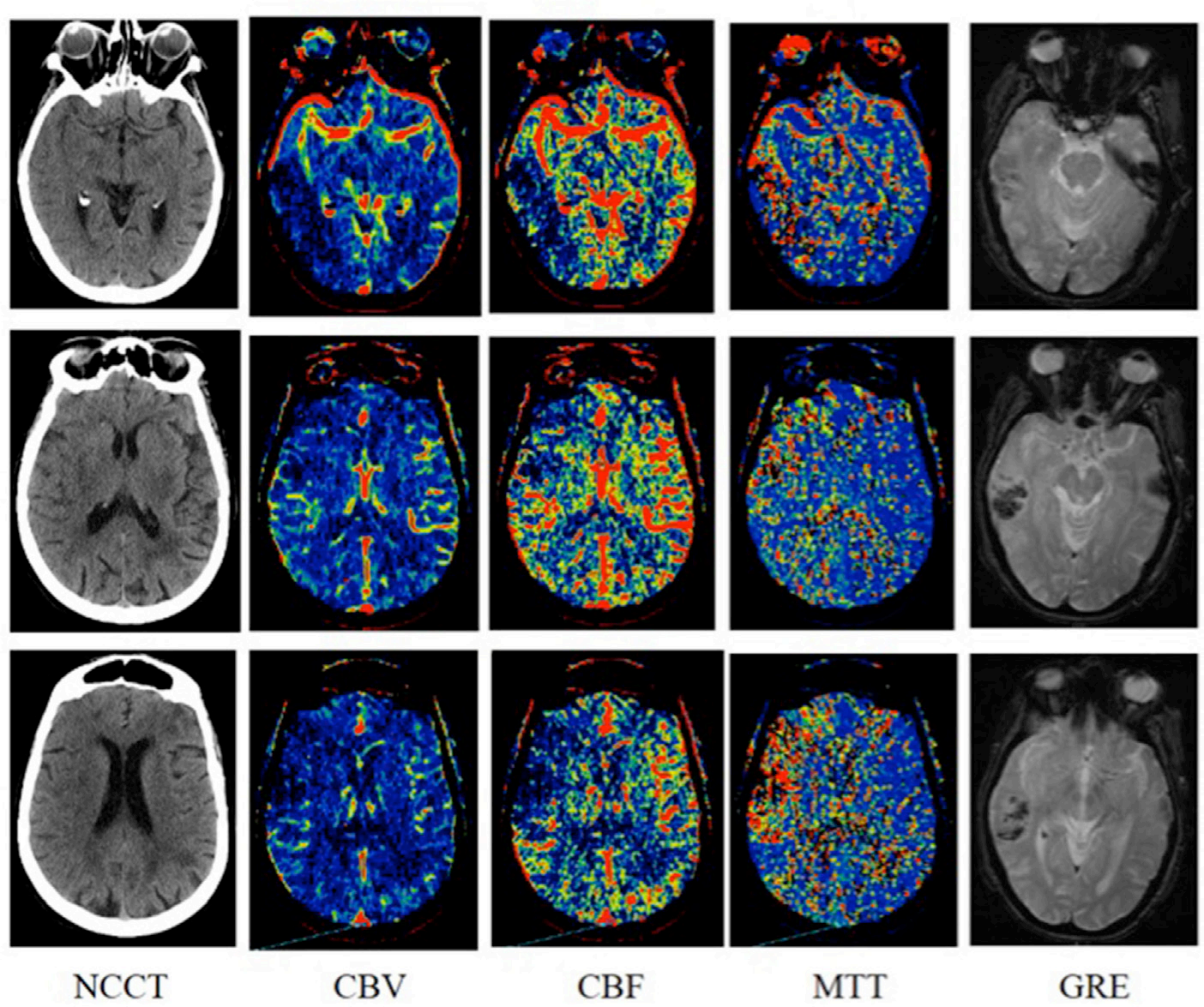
An 87-years-old woman was imaged 3 h after symptom onset with left-sided weakness. Subtle loss of gray-white matter differentiation in the right insular ribbon on non-contrast computed tomography [NCCT; Alberta Stroke Program Early Computed Tomography (CT) score (ASPECTS), 9]. Reduced cerebral blood volume (CBV; ASPECTS, 5) involving the right temporal lobe and frontal lobe in the middle cerebral artery (MCA) territory and more obvious abnormality on cerebral blood flow (CBF; ASPECTS, 4) and mean transit time (MTT; ASPECTS, 4) in the right temporal lobe, frontal lobe and insular cortex. After 7 h of symptom onset hyperintensity on diffusion-weighted imaging (DWI) in the right temporal lobe, frontal lobe, and insular cortex and hypointensity on T2*-weighted gradient echo (GRE) was identified in the right temporal lobe.
